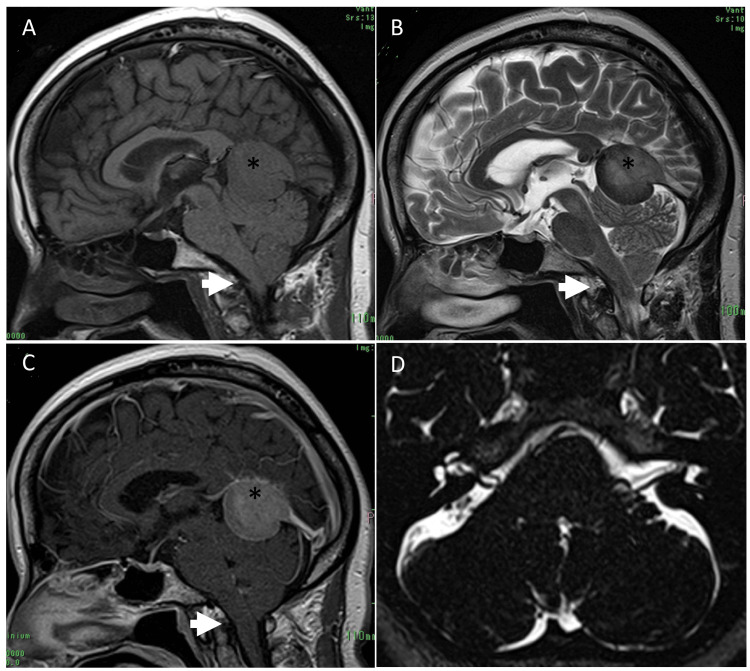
Preoperative magnetic resonance (MR) images (A, B, C) are all sagittal views, showing T1-weighted, T2-weighted, and contrast-enhanced T1-weighted images, respectively. A falcotentorial meningioma (asterisks) is lightly compressing the superior surface of the cerebellum, resulting in overcrowding at the foramen magnum (arrows). (D) is an axial view obtained with constructive interference in steady-state (CISS), demonstrating bilateral narrowing of the cerebellopontine angle cistern; however, no twisting or lateral displacement of the cerebellum or brainstem is evident.
